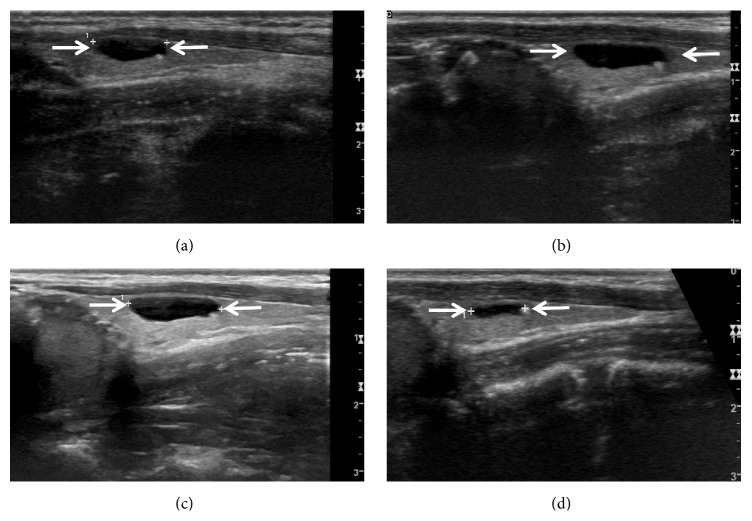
A thyroid colloid cyst with positive fluctuation in a 40-year-old woman. Longitudinal sonogram (a) shows a colloid cyst with an anechoic nodule and a comet-tail artifact in the left thyroid lobe (arrows, 11.1 mm at its largest diameter). Upon sequential US follow-ups, this colloid cyst demonstrates a gradual increase at a 1-year (b) (arrows, 13.0 mm at its largest diameter) and a 2-year (c) (arrows, 13.8 mm at its largest diameter) US follow-ups, but it shows a moderate decrease on 3-year follow-up US (d) (arrows, 8.4 mm at its largest diameter).
